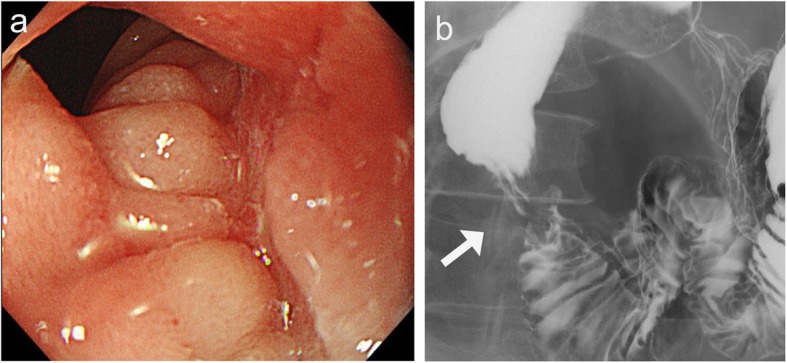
a, b Upper gastrointestinal endoscopy and X-ray radiography after chemotherapy also showed the decreased size of the ulcerative duodenal tumor (arrow)
